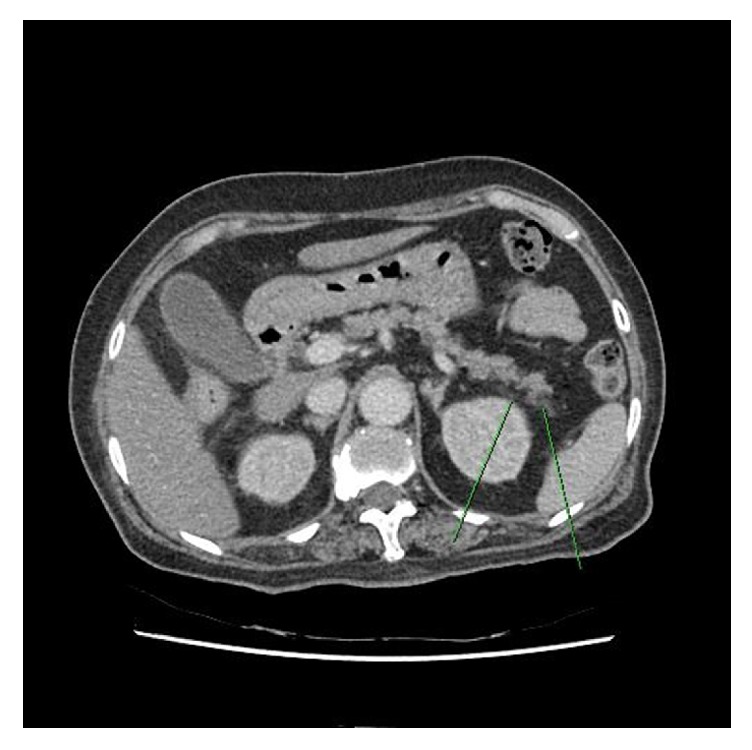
Abdominal computer tomography (CT) scan shows acute pancreatitis. Contrast enhanced abdominal CT scan shows fat infiltration in the tail portion of the pancreas parenchyma, suggesting acute pancreatitis grade C.
